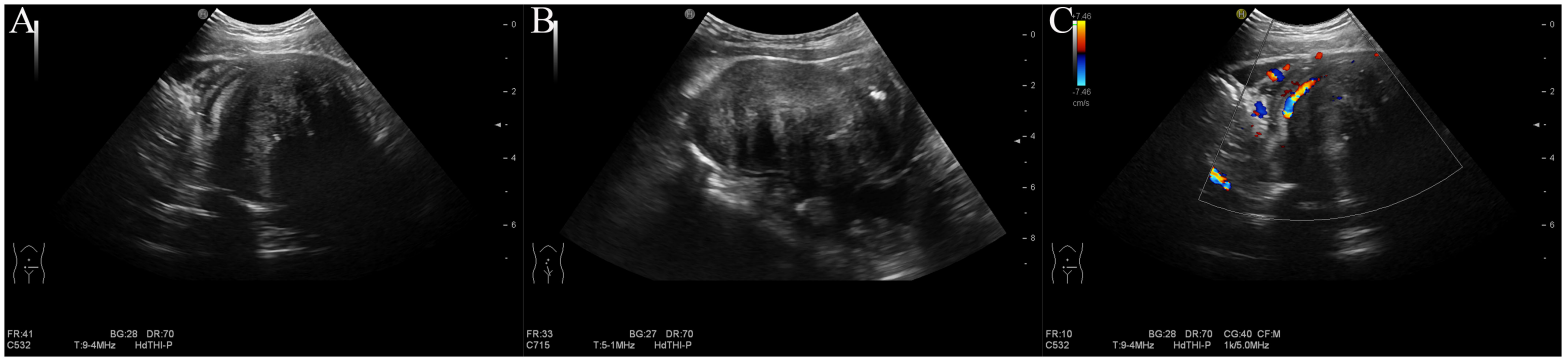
Conventional ultrasound. (A) Transverse view: a hypoechoic mass in the lower abdomen with slightly hyperechoic fibrous strands and attenuation. (B) Longitudinal view of the mass. (C) Color doppler imaging reveals blood flow signals within and surrounding the mass, originating from the small intestine wall and mesentery.
